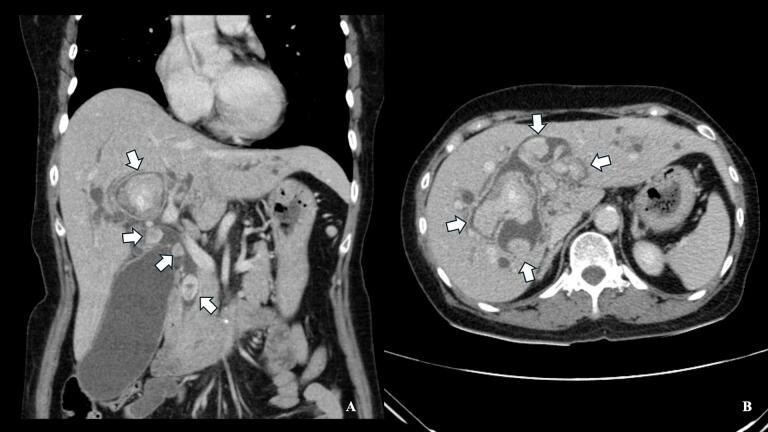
Computed tomography findings in recurrent pyogenic cholangitis. (A) Coronal and (B) axial contrast-enhanced CT images of a 65-year-old female with recurrent pyogenic cholangitis. Multiple hyperdense structures (white arrows) are visible within diffusely dilated intrahepatic ducts and common bile duct, consistent with pigmented stones. Note the widespread biliary dilatation, a hallmark of recurrent pyogenic cholangitis.
